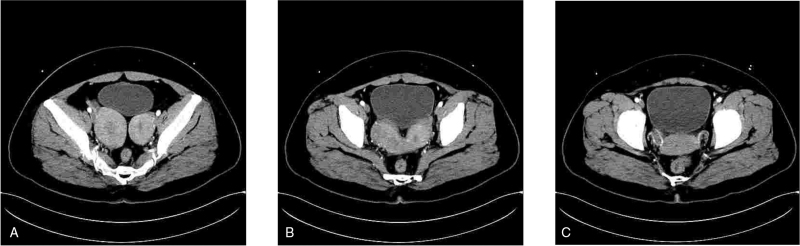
CT findings of the uterus. (A) CT shows bilateral enlargement of the uterine body. (B) Bilateral uterine fusion and adhesion to the bladder wall. (C) Bilateral cervix and bilateral uterine arteries. CT = computed tomography.
